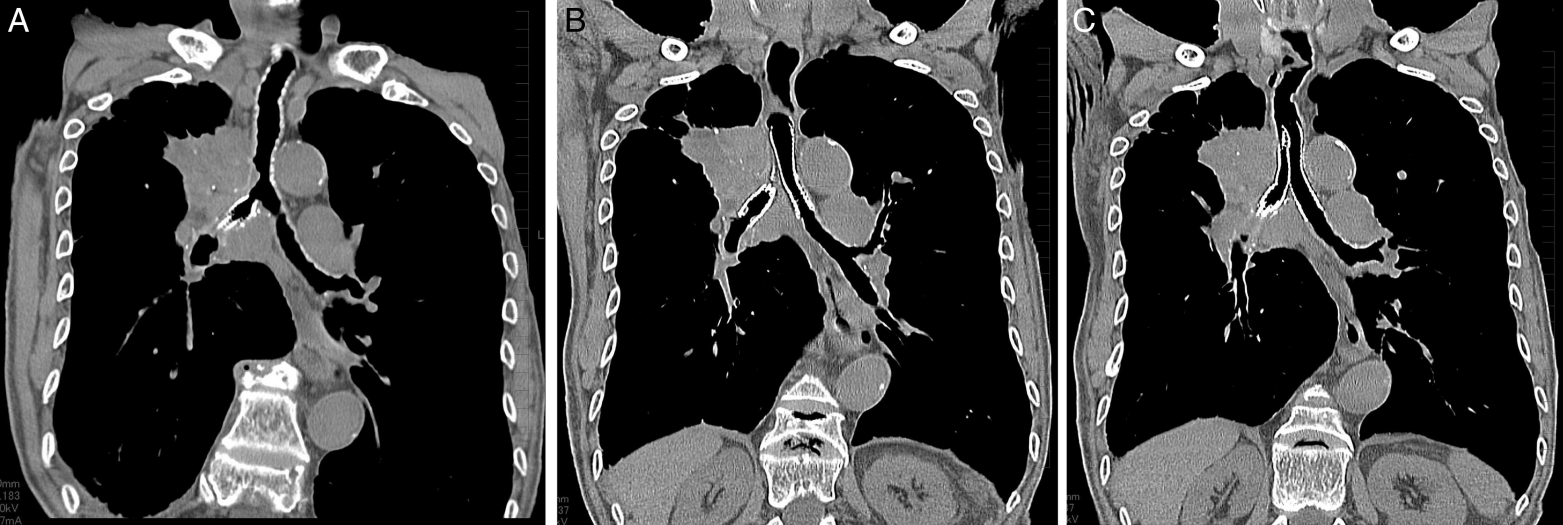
The representative chest coronal computed tomography images are shown before (A) and 7 days after (B, C) the ‘side‐by‐side’ method of bilateral self‐expandable metallic stents (SEMSs) in case no.2. Severe stenosis was observed in the right mainstem bronchus, which had already been treated with an self‐expandable metallic stent (SEMS) as well as the lower trachea and the orifice of the left mainstem bronchus (A). Bilateral SEMSs were patent and adjacent to each other (B, C), and each of the proximal end was in line with each other without migration (C) 14 days after the procedure.
